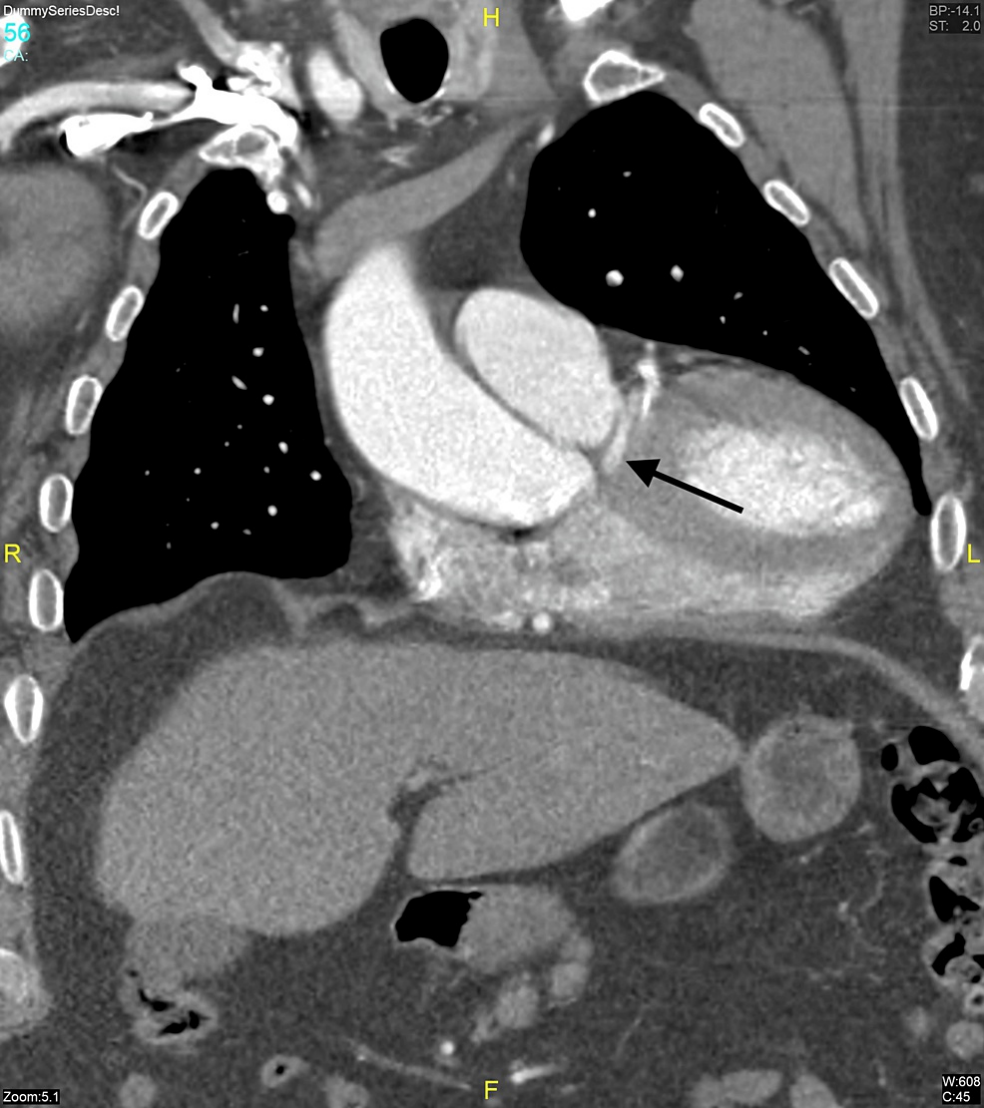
CT angiography (coronal view) showing the septal course of the LMCA (black arrow) LMCA: left main coronary artery
